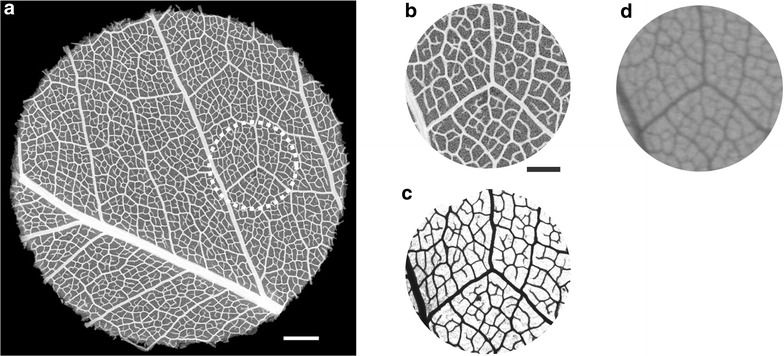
Very high-resolution imaging (virtual 2D) of leaf venation using micro CT. a Micro CT image of a leaf fragment of *Burkea africana* (Fabaceae); the circle refers to the enlarged view in b. b Detail of leaf venation with full resolution of minor veins. c leaf venation image from chemical leaf clearing; here, the same area as in b is shown. d image from 2D X-ray with 7 µm resolution. Scale 1 mm (a) or 0.5 mm (b–d)
